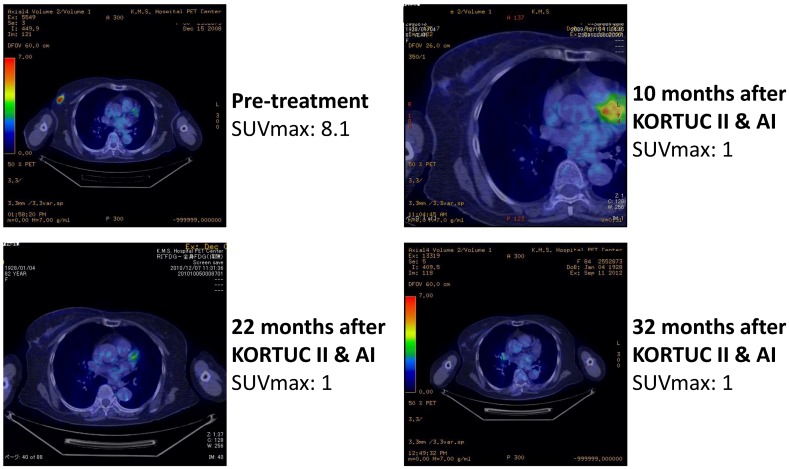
Case 18. 80 years old female patient with right breast cancer (cT2N1M0, ER: +, PgR: +, HER-2: ++ [FISH: -]) treated with radiosensitization (KORTUC II) and without systemic chemotherapy. Left-upper: PET-CT image at pre-treatment. Tumor diameter is 25 mm and SUV-max value is 8.1; Right-upper: PET-CT image at 10 months following KORTUC II. Tumor is not recognized and SUV-max value on the region is approximately 1; Left-lower: PET-CT image at 22 months following KORTUC II. Tumor is not recognized and SUV-max value on the region is approximately 1; Right-lower: PET-CT image at 32 months following KORTUC II. Tumor is not recognized and SUV-max value on the region is approximately 1.
